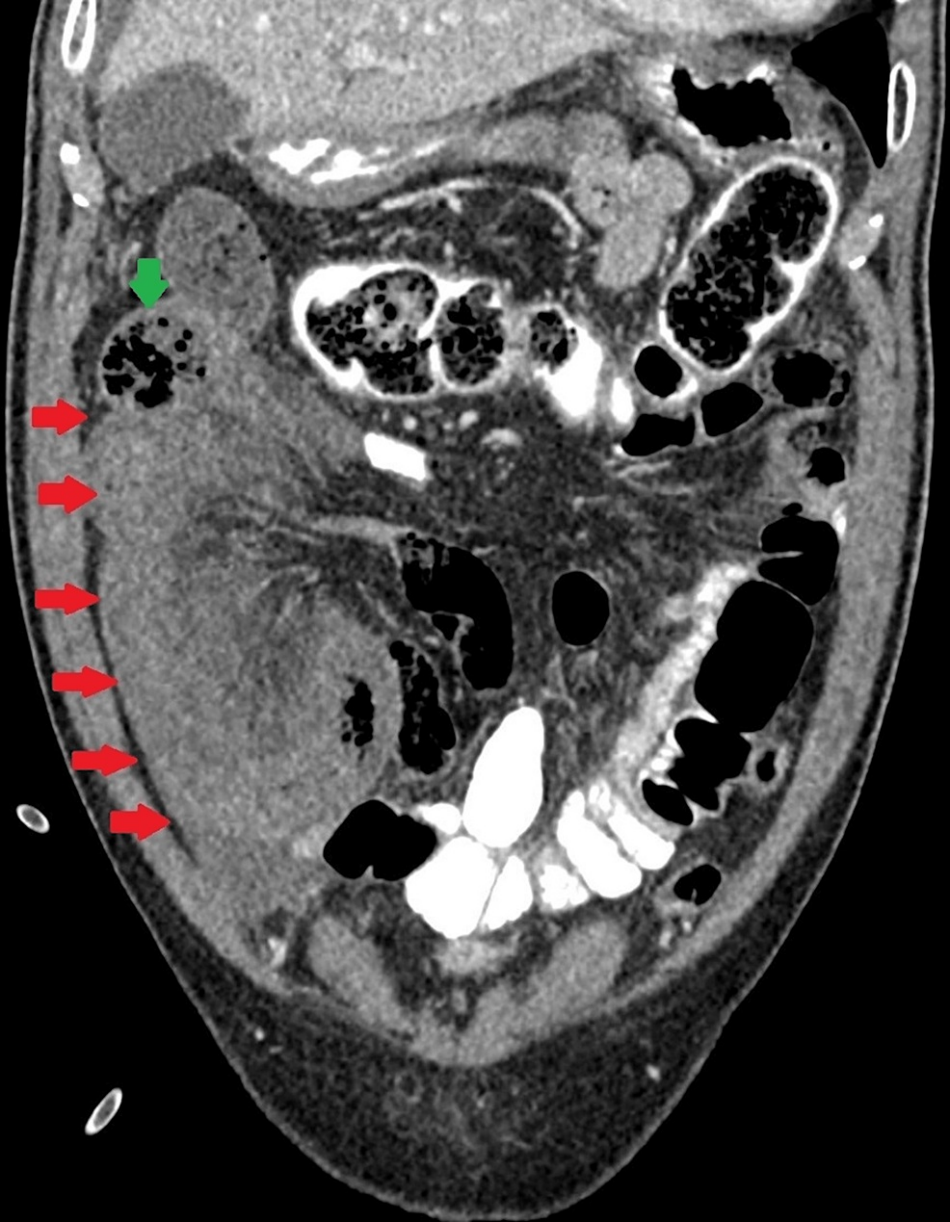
Coronal view of a computed tomography (CT) scan revealing C-shaped clustered loops of the ileum (red arrows) converging medially. Presence of small bowel feces sign (green arrow).
